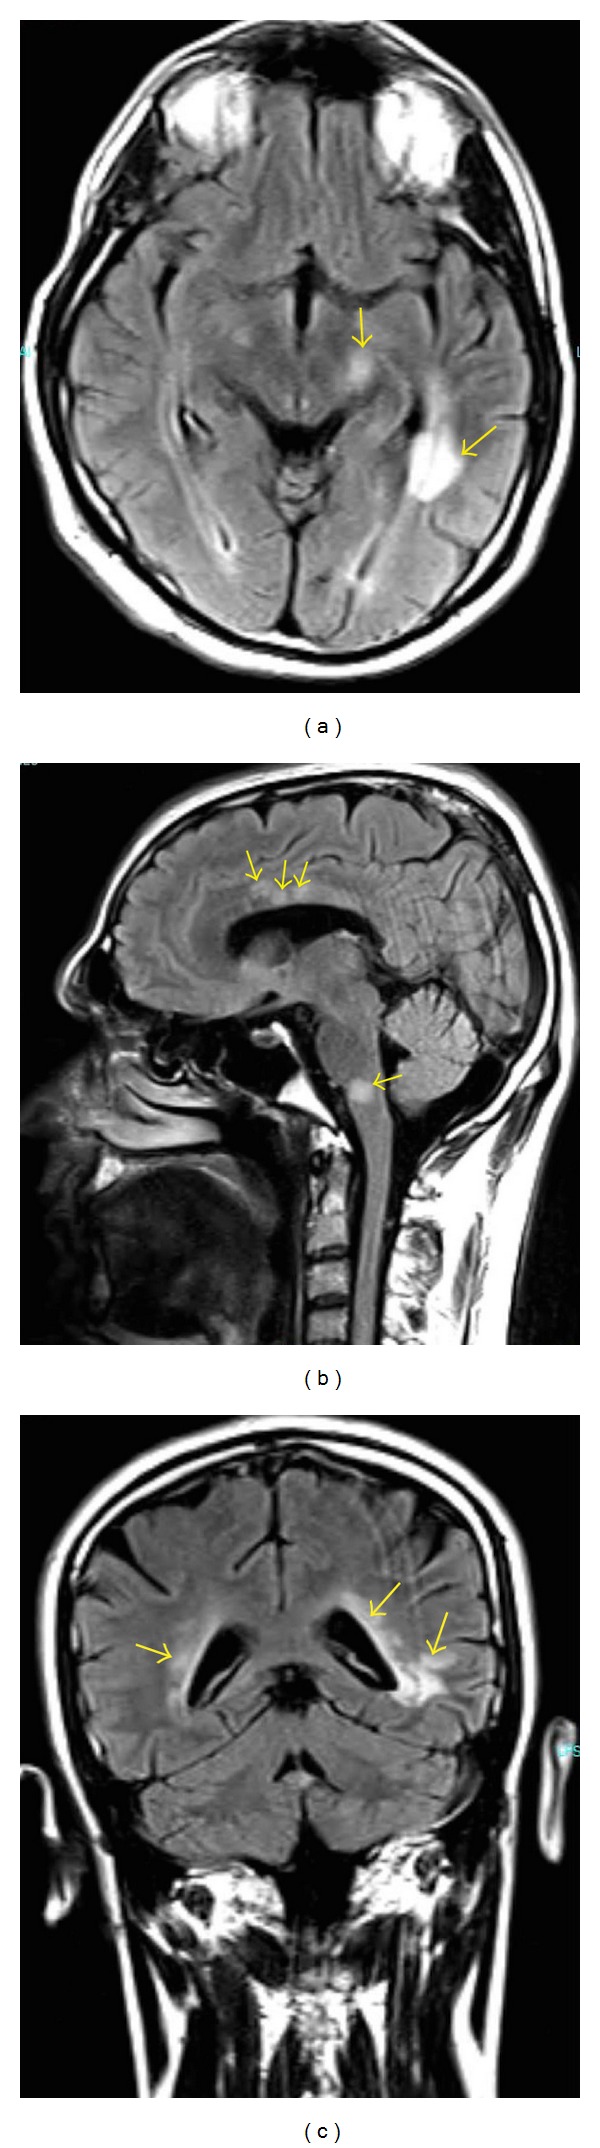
Cranial MRI showing (a) axial, (b) sagittal, and (c) coronal T2-weighted FLAIR views of the demyelinating lesions in the bilateral periventricular, juxtacortical, and infratentorial regions (arrows).
